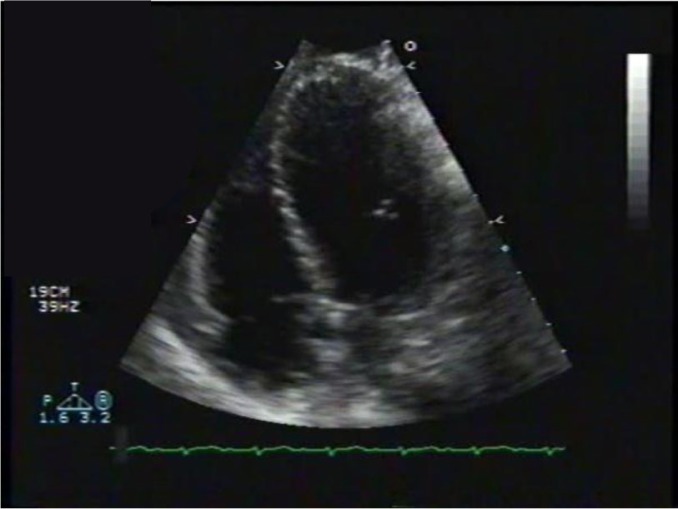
Echocardiogram, pre-diastolic.
